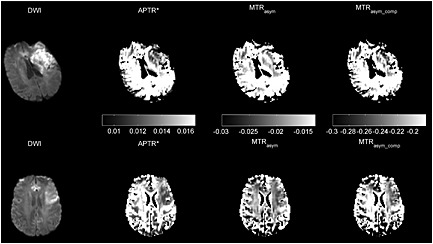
Diffusion‐weighted imaging (DWI) (*b* = 1000 s/mm^2^) and processed amide proton transfer (APT) results (APTR*, MTR_asym_ and MTR_asym_comp_) from representative patients: top row, Patient 3; bottom row, Patient 5. Tissue masks were used to remove the non‐tissue areas, such as cerebrospinal fluid (CSF); thus, the scale bar does not reflect the quantified APT effect in these areas.
